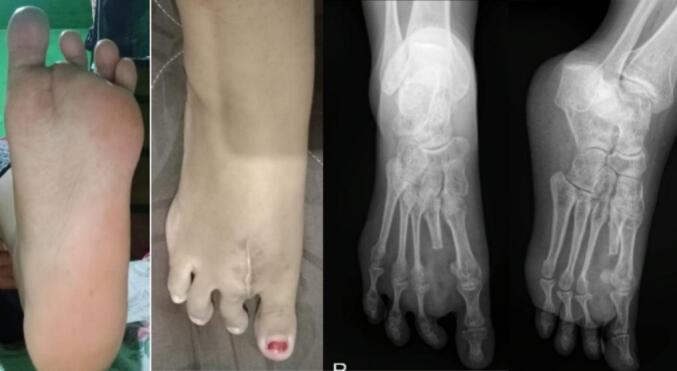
Follow up 4 months post surgery surgery. Postoperative x-rays after performing the second ray amputation showed no sign of recurrence.
